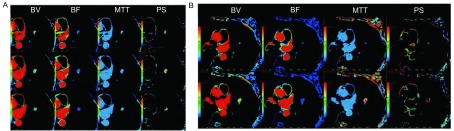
女性，34岁，左肺上叶腺癌，Ⅳ期，最大径约2.1 cm。患者第二次检查时，定位扫描与动态增强扫描的屏气程度不一，致扫描未完全覆盖肿瘤，CTP参数值变化较大，第二次PS值较首次增大了95%，MTT增大了153%，BV、BF值也有较大变化，分别增大了28%及降低了27%。A为第一次CTP，BV=3.46 mL/100g，BF=87.47 mL•min^-1^•100g^-1^，MTT=3.11 s，PS=11.75 mL•min^-1^•100g^-1^；B为第二次CTP，BV=4.44 mL/100g，BF=64.10 mL•min^-1^•100g-^-1^，MTT=7.38 s，PS=22.95 mL•min^-1^•100g^-1^。 A female, 34 years old, stage Ⅳ, had a adenocarcinoma in the left upper lobe, and the diameter was 2.1 cm. When the second examination was performed, CTP didn't cover the whole tumor, owing to the different breath holding. The CTP parameters varied much: the second PS value was 95% increasingly, the MTT value was 153% increasingly, the BV value was 28% increasingly, and the BF value decreasingly 27%, compared with the first CT scan. In the first CTP, the BV value was 3.46 mL/100g, the BF value was 87.47 mL•min^-1^•100g^-1^, the MTT value was 3.11 s, and the PS value was 11.75 mL•min^-1^•100g^-1^ (A). In the second CTP, the BV value was 4.44 mL/100g, the BF value was 64.10 mL•min^-1^•100g^-1^, the MTT value was 7.38 s, and the PS value was 22.95 mL•min^-1^•100g^-1^ (B).
